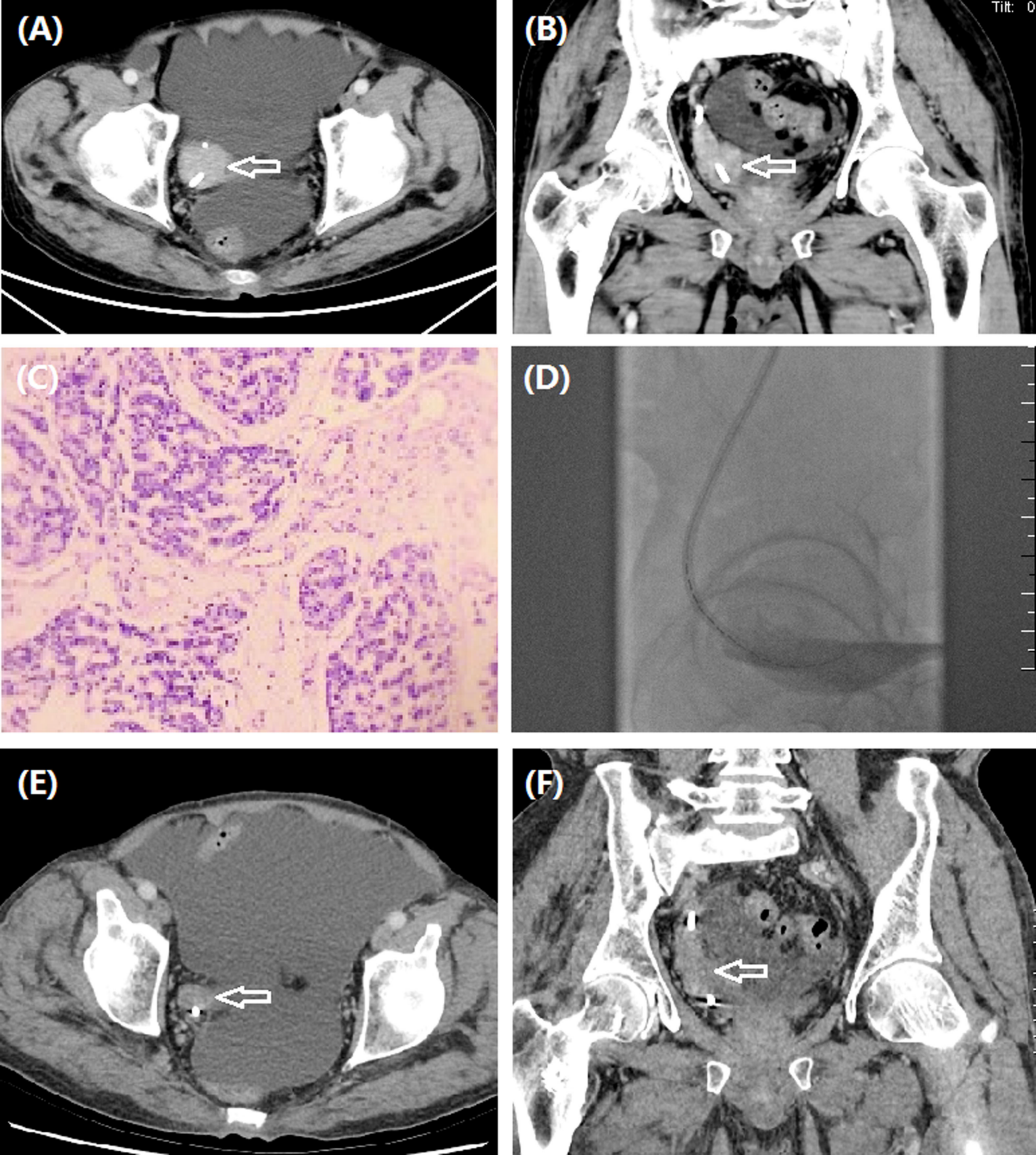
A 61-year man treated by iodine-125 seed strand for right ureteral carcinoma. (A, B) CT revealed ureteral carcinoma (arrow) of right lower ureter. (C) Pathology was confirmed to be ureteric papillary urothelial carcinoma with tumor invasion in the local lamina propria. (D) Iodine-125 seed strand with 15 seeds was inserted in the occlusive segment. (E–F) Stable tumor (arrow) was detected by CT after 6.0 months.
